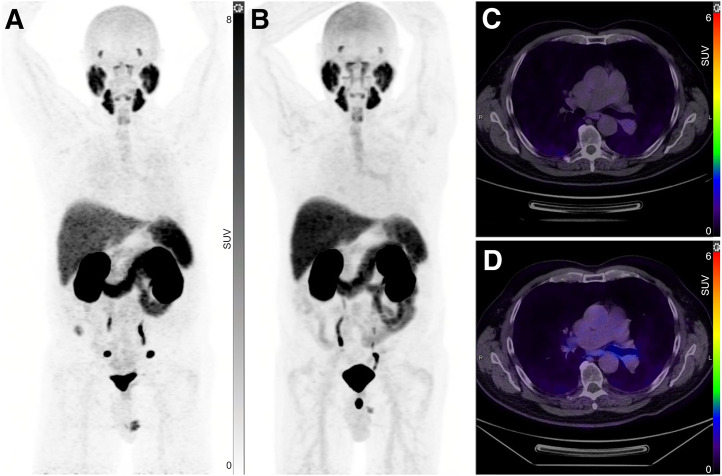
Baseline ^68^Ga-PSMA-11 and 1 y posttherapy ^18^F-DCFPyL images for same patient. (A and C) ^68^Ga-PSMA-11 PET maximum-intensity projection (A) and fused axial PET/CT image (C) showing minimal tracheal uptake 60 min after injection. (B and D) ^18^F-DCFPyL PET maximum-intensity projection (B) and fused axial PET/CT image (D) showing relatively higher tracheal uptake 90 min after injection.
